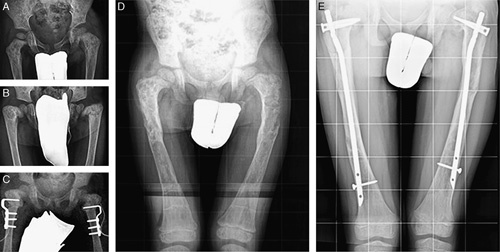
A, Patient with severe bilateral coxa vara with a painful waddling gait; bilateral valgus hip osteotomy (B) was performed. C, Standing radiograph at 6 years of age with type 4 femoral deformities. D, At 10 years of age, 4 years after bilateral femoral intramedullary nailing with adult humeral nails, bilateral coxa vara recurrence, and relative shortening of the nails with growth were evident (E).
